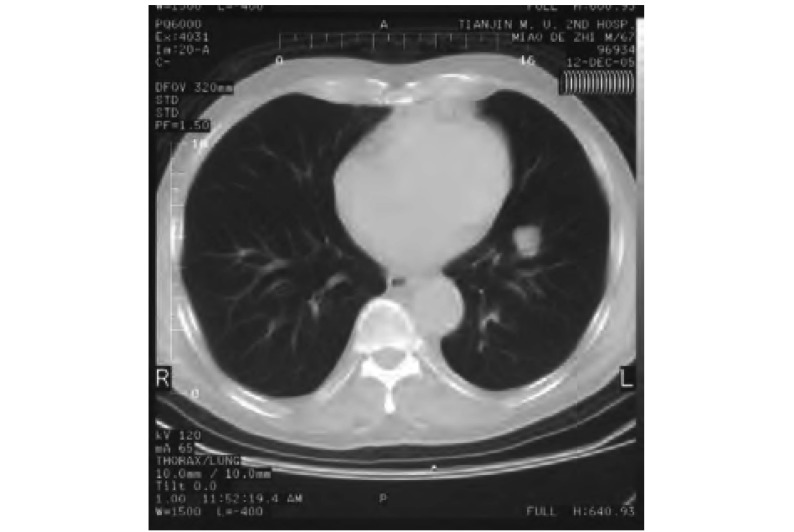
术前胸部CT，LCNEC病灶位于左肺上叶前段 The chest CT scan of the LCNEC preoperative, lesion locate at anterior segmental of the left upper lobe
